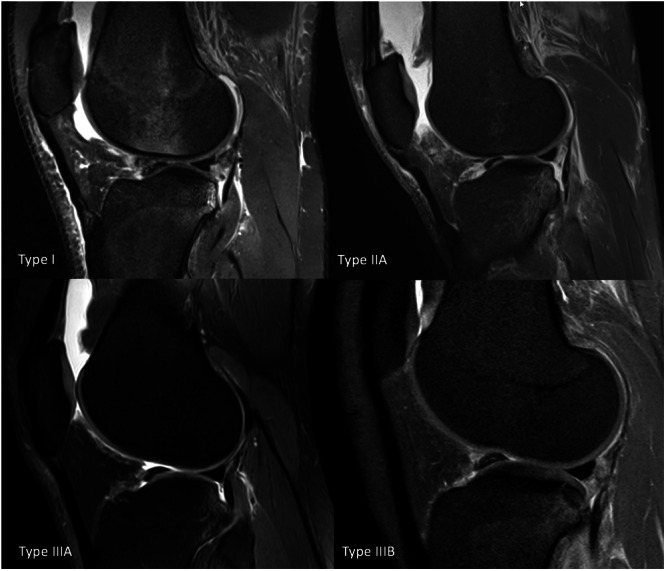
Representation of posterolateral tibial plateau impaction fracture types (according to Bernholt et al. [2]) in the study population examined, in sagittal T2‐weighted magnetic resonance imaging (MRI) scans.
